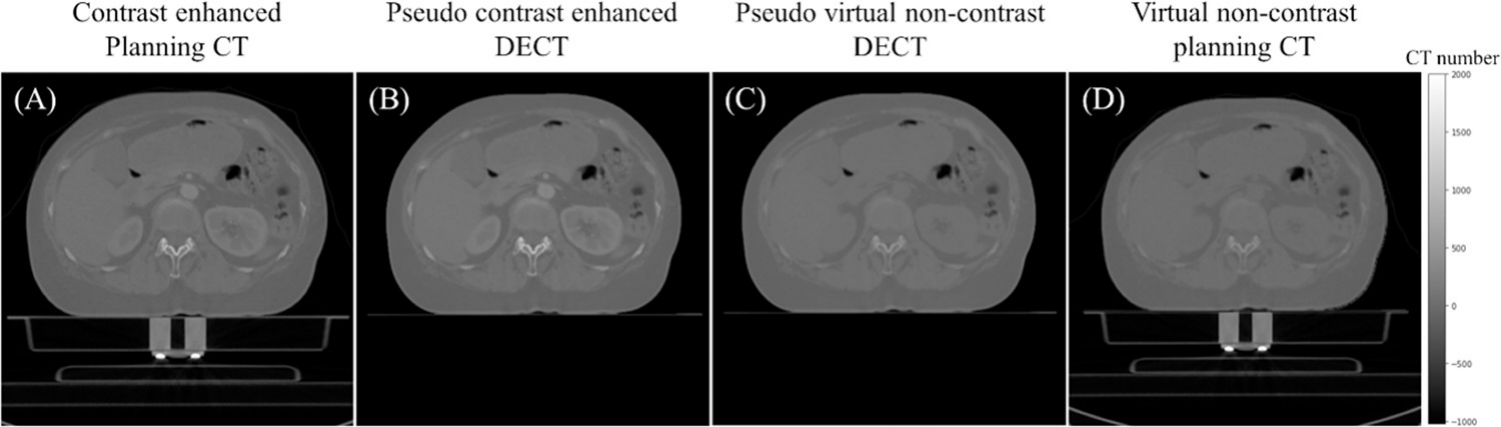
Conversion between pseudo DECT and pCT. CE-pCT (A), pseudo DECT (B), pseudo VNC DECT (C), and VNC-pCT (D). To ensure the anatomy is clearly visible, CE-pCT and VNC-pCT were enlarged to match the body size of pseudo DECT and pseudo VNC DECT. CE-pCT, contrast enhanced planning computed tomography; DECT, dual-energy computed tomography; pCT, planning CT; VNC DECT, virtual non-contrast dual-energy CT; VNC-pCT, virtual non-contrast planning computed tomography.
